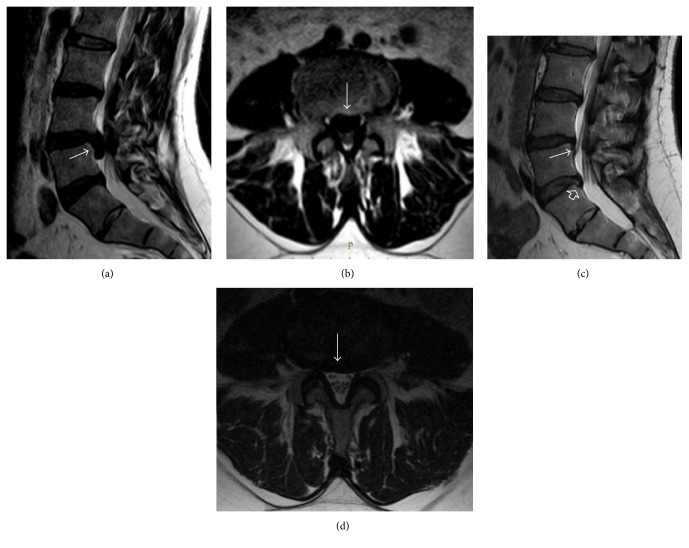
(a) A huge extruded disc herniation that migrated caudally (white arrow) at L4-5 level on T2 weighted sagittal lumbar MR images. (b) Axial view of the same disc, extruded disc occupying nearly half of the spinal canal. (c and d) The extruded disc has disappeared completely (white arrows) in sagittal and axial MR images after three years; the height of the disc space slightly decreased at L4-5 level and there was no sign of compression. The protrusion of L5-S1 disc (arrow head) that will be obvious in the left sagittal images.
